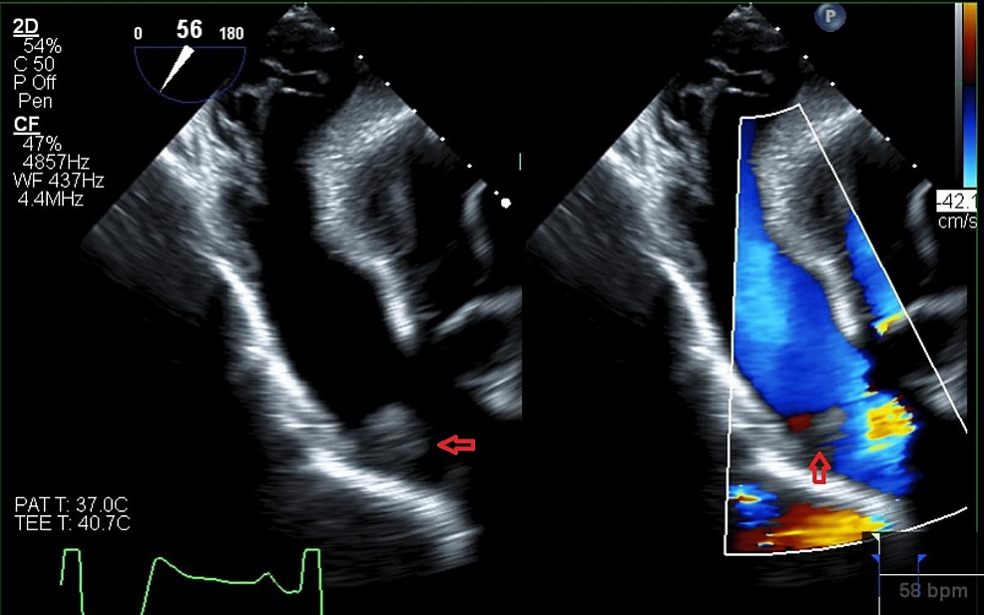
Transgastric right ventricle outflow view on transesophageal echocardiogram. No significant systolic flow acceleration on the color doppler (left panel). Suspected myxoma on the pulmonic valve leaflet (red arrows)
